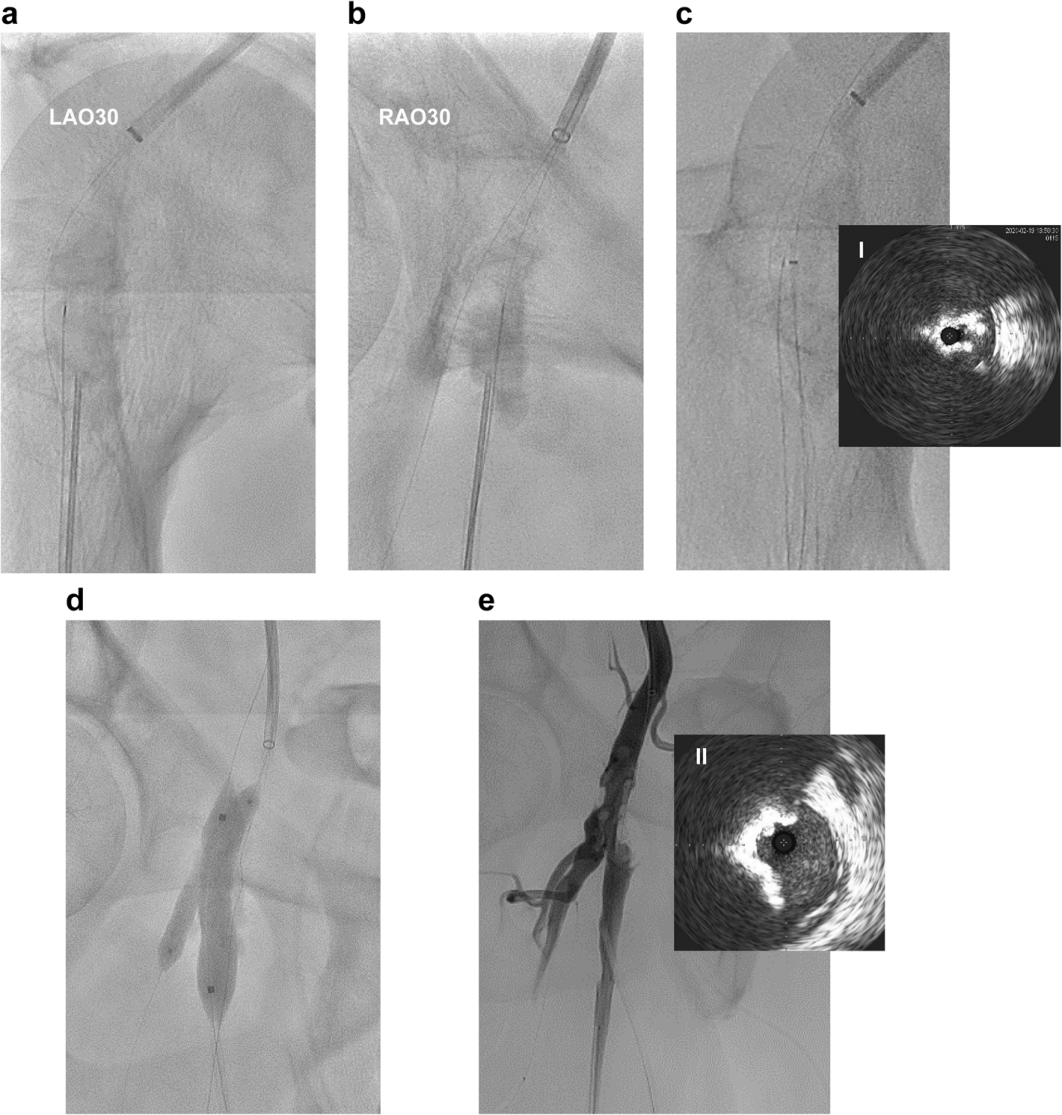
Angiography and intravascular ultrasound (IVUS) imaging. A, B Outer cylinder of 20G long needle (introducer needle, Medikit, Tokyo, Japan), the tip facing the center of the calcified plaque was checked by 2 perpendicular views in fluoroscopy. C Crosser. C-I) IVUS images showing the route created through the calcified plaque. D A kissing balloon inflation was performed with a 10 × 40 mm scoring balloon (NSE PTA; Nipro, Osaka, Japan) for SFA and with a 6 × 40 mm semi-compliant balloon (Sterling; Boston Scientific, Natick, MA) for DFA. E) final angiography. E-II) IVUS showing the lumen area gained
